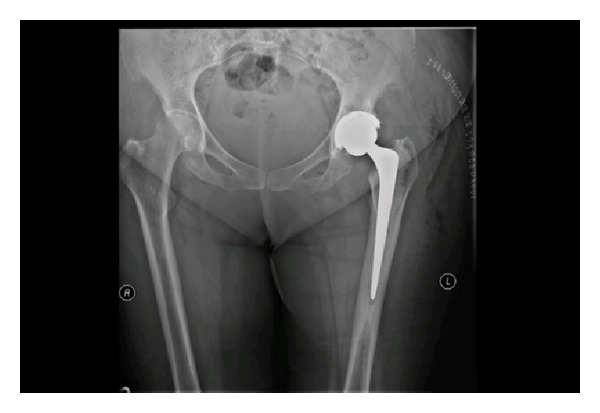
Plain radiograph taken on day 1 after initial left total hip arthroplasty.
